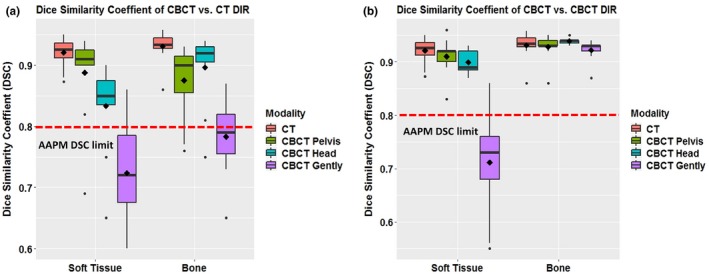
Accuracy of deformable image registration measured with the Dice similarity coefficient (DSC). Box‐and‐whisker plot shows the accuracy of deformable image registration measured with the DSC. The thick horizontal lines represent the median. The diamond indicates the mean value. The lower and upper boundary correspond to the first and third quartiles (the 25th and 75th percentiles). The whiskers show the maximum and minimum values, up to 1.5 times the inter‐quartile range. The dots indicate outliers. (a) Cross‐modality image registration (CBCT vs CT, also showed CT vs CT as a reference). (b) Same‐modality image registration (CBCT vs CBCT, also showed CT vs CT as a reference). CBCT, cone‐beam computed tomography.
